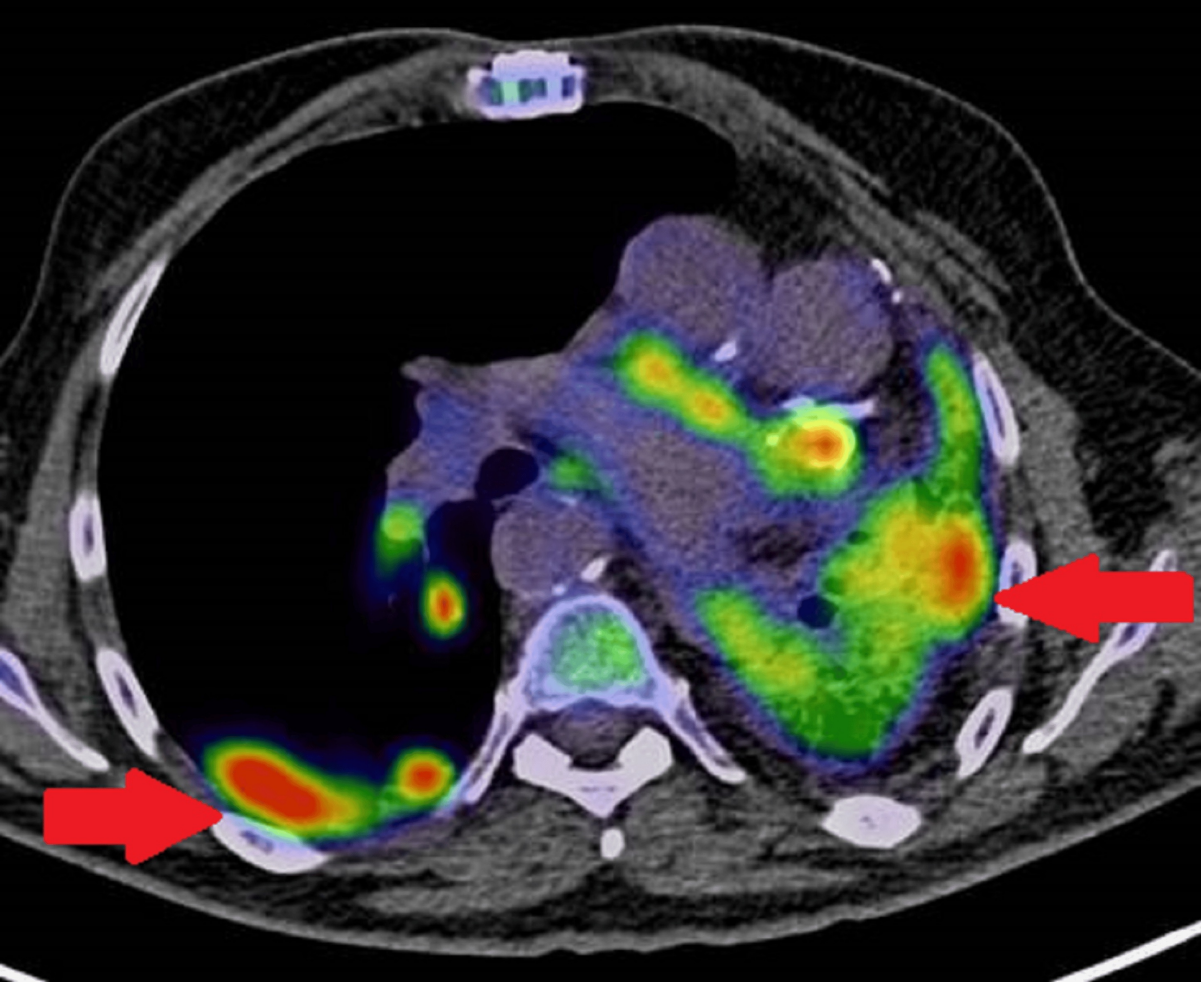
Axial positron emission tomography/computed tomography (PET/CT) image. The image demonstrates multifocal fluorodeoxyglucose (FDG) uptake in the lower lobes, greater on the left than the right (red arrows), corresponding to areas of increased ground-glass opacities noted on the diagnostic chest CT.
